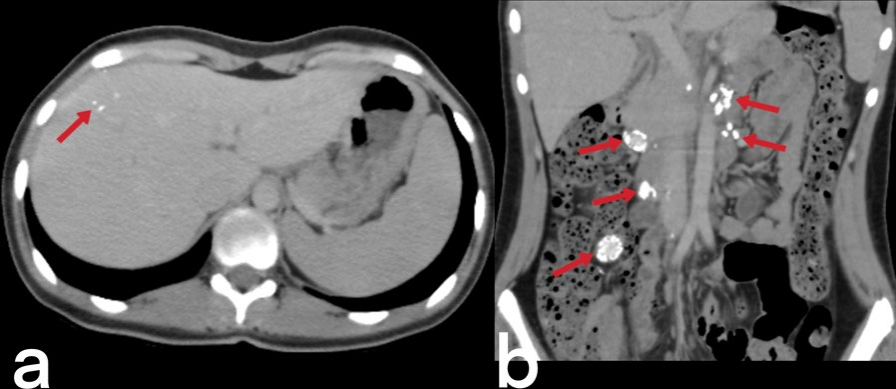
Transverse abdomen CT image (a) of a 17-year-old female with known TB. Coarse calcifications in the liver parenchyma suggestive of TB sequela are noted. Coronal reformatted CT image of the same patient (b) reveals multiple lymphadenopathies with peripheral calcifications
